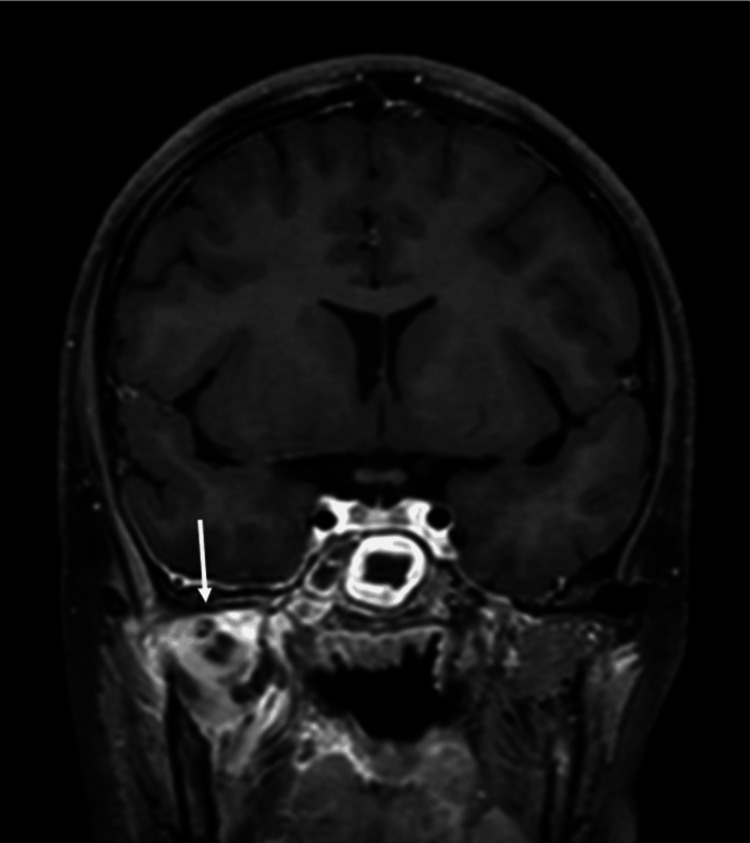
Second hospital admission. Coronal slice MRI head with contrast showing a 2.4 x 2.2 x 3.3 cm multiloculated abscess in the right masticator space
